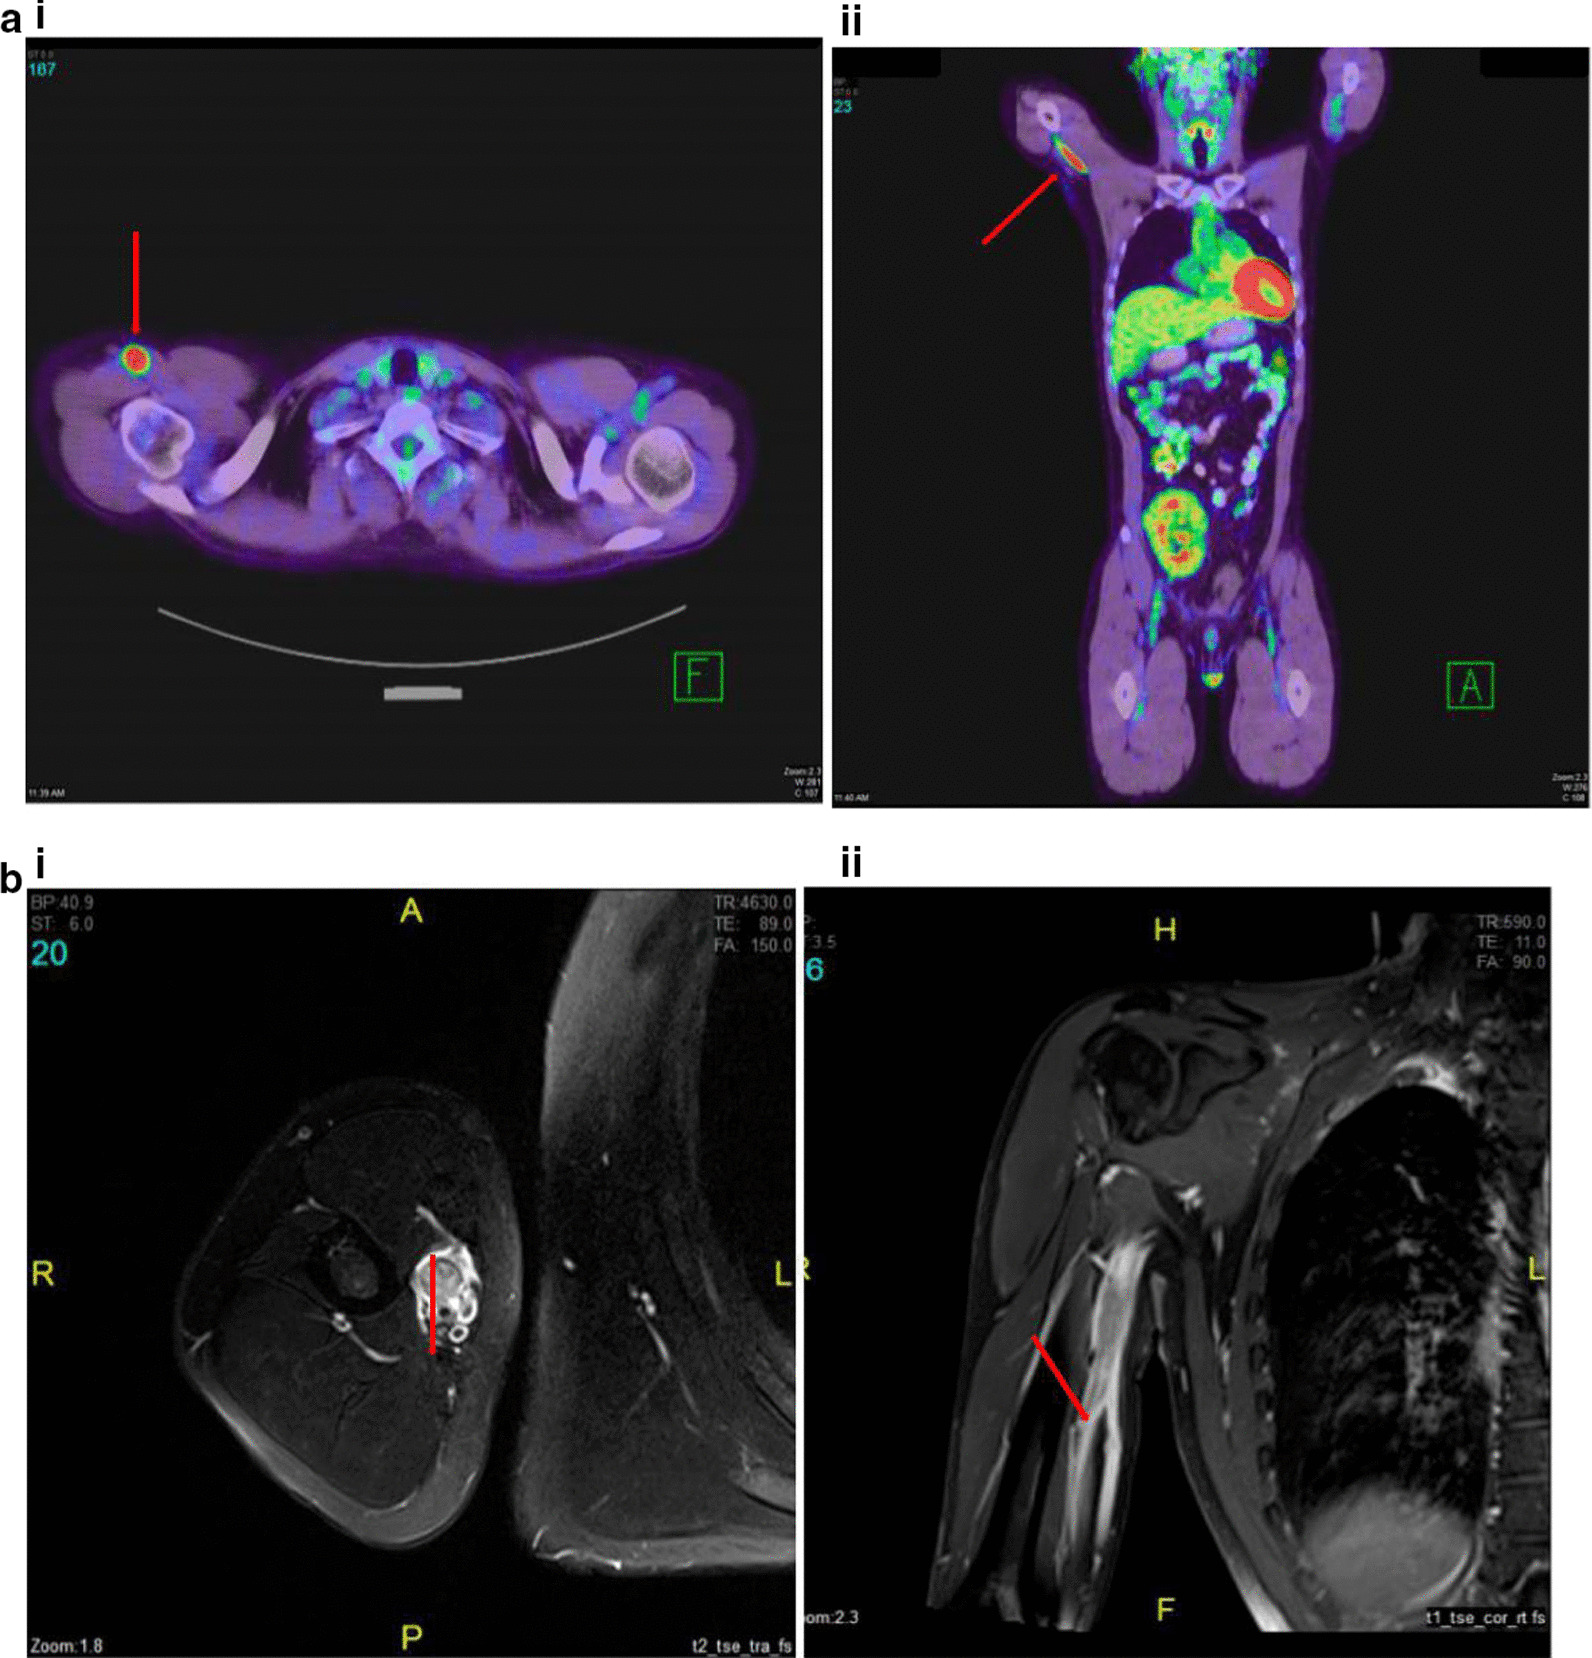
Case 1, radiological findings. a FDG PET-CT. Axial (i) and coronal (ii) planes show abnormally avid activity in the anatomical location of the right upper arm median nerve (arrows). b MRI axial T1-weighted post-contrast fat-saturated image (i). Coronal T1-weighted post-contrast fat-saturated image (ii). The right upper arm median nerve (arrows) is enlarged and displays abnormal signal intensity (low and high T1- and T2-weighted images, respectively) and abnormal post-contrast heterogeneous enhancement (b, i–ii)
